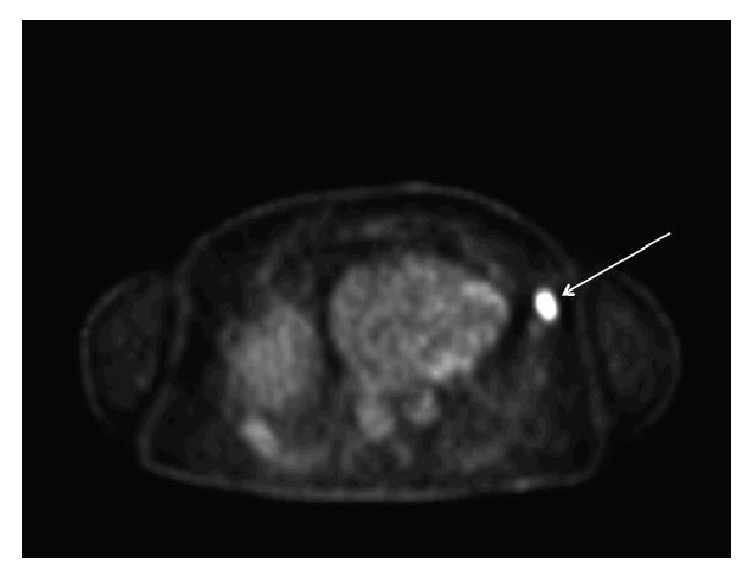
PET scan identifies intensely hypermetabolic nodular lesion on the left lateral chest wall inferior to left axilla, highly suspicious for malignancy (max SUV 12.5).
